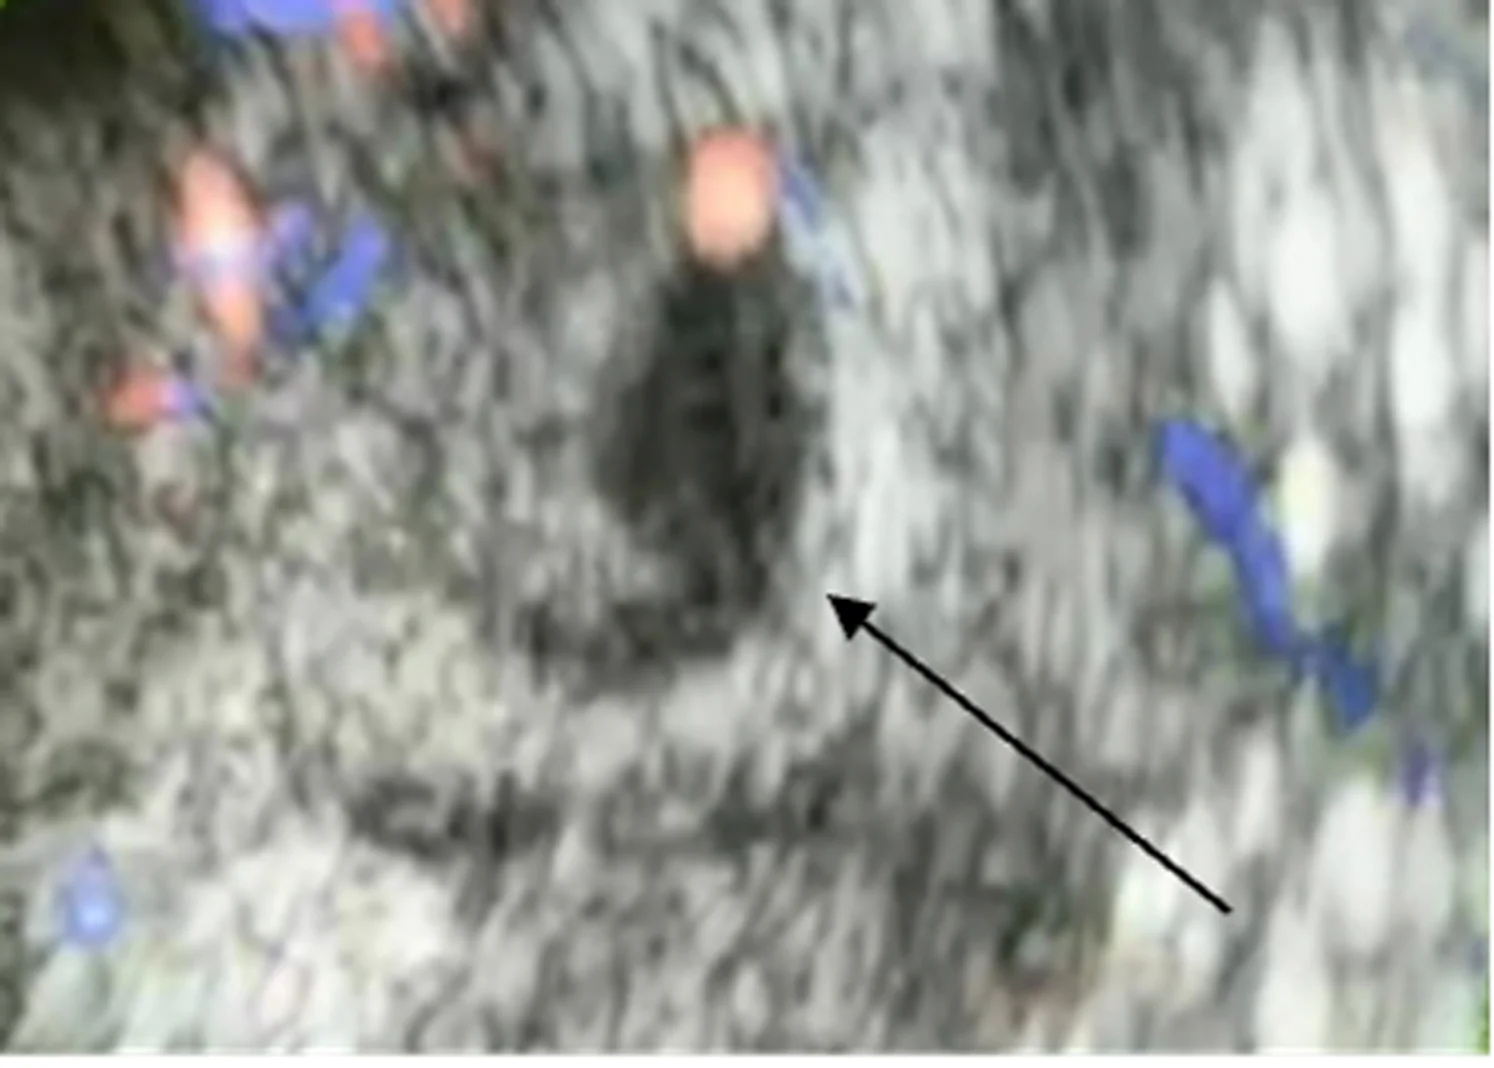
Endobronchial ultrasound showing vascular structure (black arrow) with turbulent, swirling flow concerning for a pseudoaneurysm.
